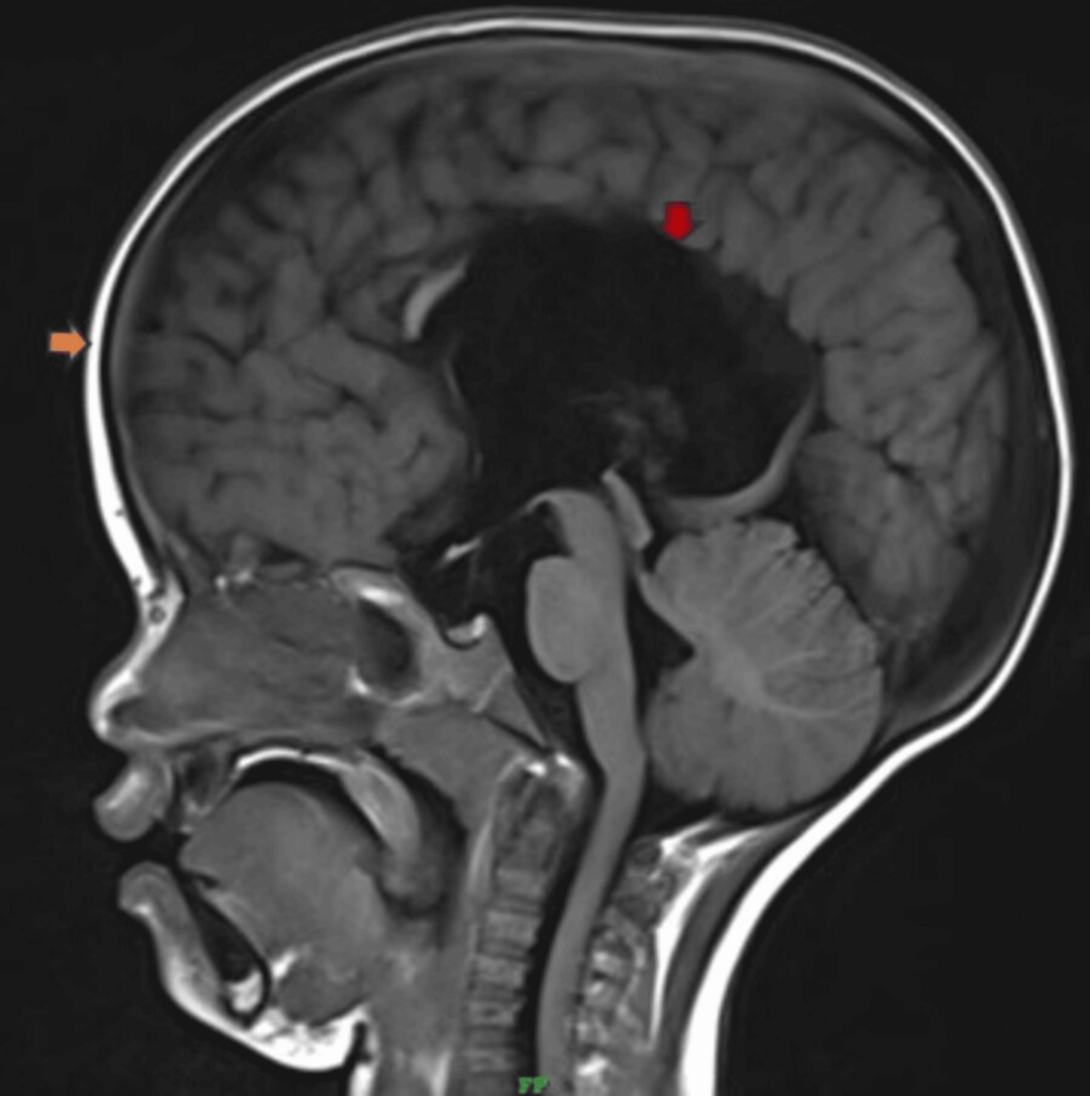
MRI T1WI sagittal image The image demonstrates partial dysgenesis of the corpus callosum (red arrow) and dolichocephaly (yellow arrow) MRI: magnetic resonance imaging; T1WI: T1-weighted imaging
